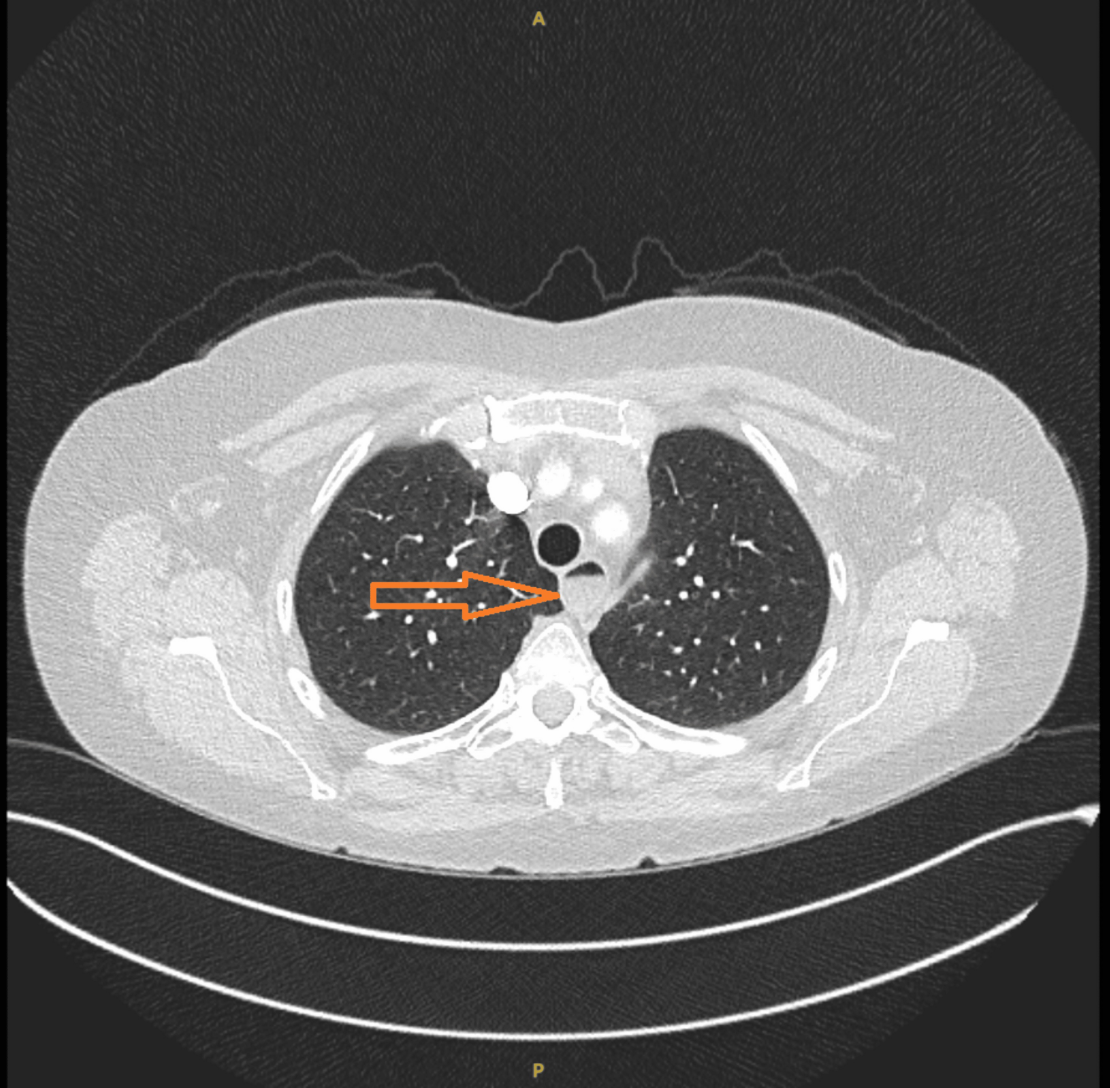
Axial contrast-enhanced CTPA showing normal-calibre pulmonary arteries with no filling defects. Mild oesophageal dilatation with retained fluid is noted CTPA: computed tomography pulmonary angiography
